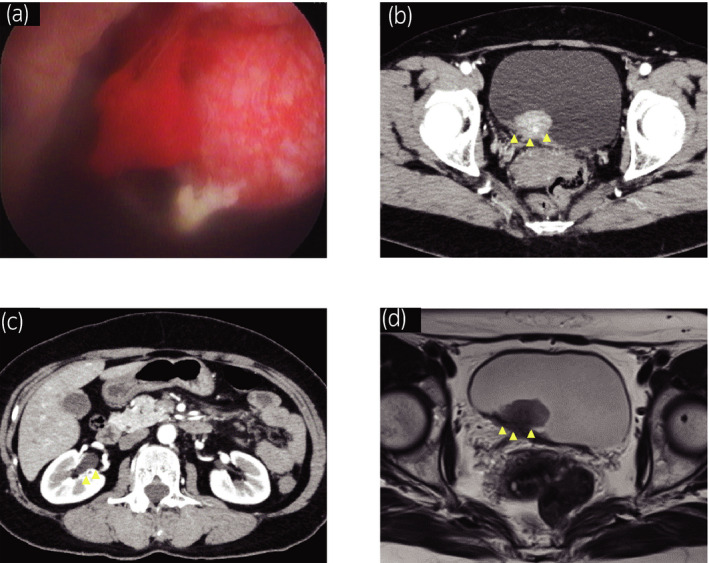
Clinical and radiological findings before TURBT. (a) Cystoscopy showed a sessile non‐papillary bladder tumor adjacent to the right ureteric orifice. (b) Abdominal CT with contrast showed a 20‐mm bladder tumor at the right posterior bladder wall and (c) Grade 1 right hydronephrosis. (d) Abdominal MRI showed a 20‐mm bladder tumor suspected for invasion to the muscular layer at the right posterior bladder wall on T2 weighted image.
